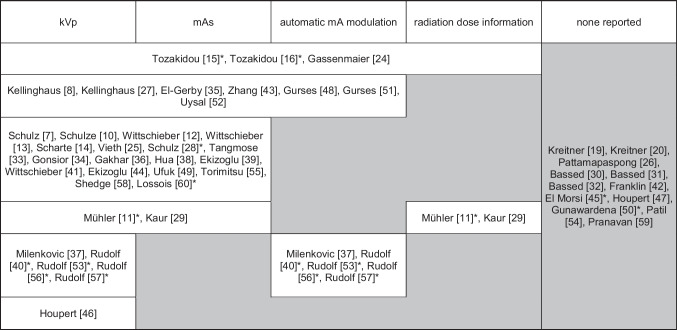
Reported CT scan parameters and dose parameters
